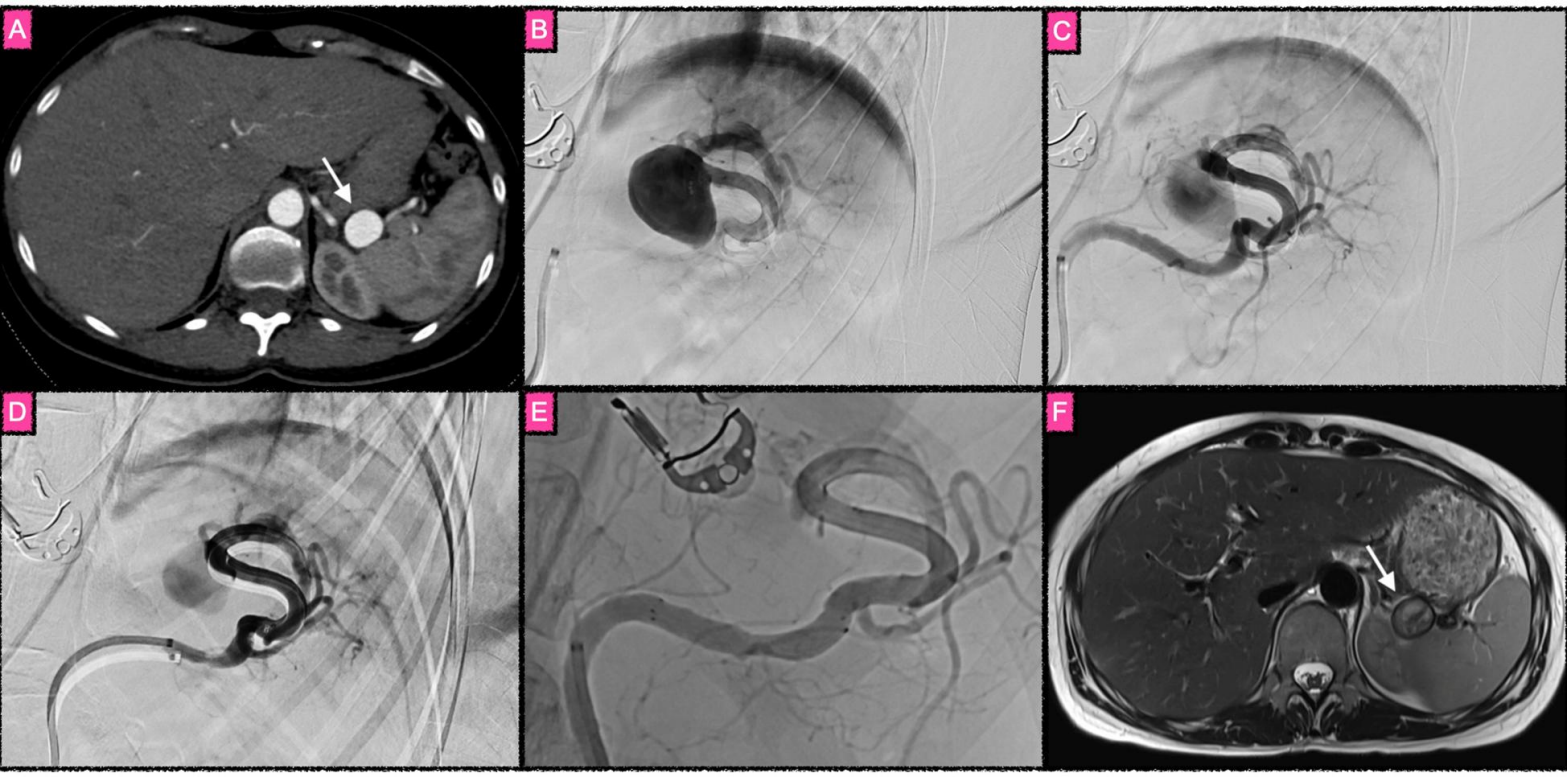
Treatment of a giant main stem aneurysm of the splenic artery with four overlapping flow diverter stents, equipped with antithrombogenic HEAL coating under prasugrel monotherapy. Given the large size of the aneurysm, the risk for delayed rupture after flow diversion–a rare but potentially fatal complication most likely occurring secondary to excessive thromboinflammation–was estimated to be comparatively high. Therefore, antiplatelet medication (prasugrel only) was reduced, based on the use of HEAL-coated FDS, which are significantly less thrombogenic and less proinflammatory. (A) Axial section of a computed tomography angiography in the arterial phase with the aneurysm (white arrow) in close proximity to the spleen. (B) Early phase of a superselective catheter angiography of the splenic artery after implantation of two FDS, with two adjacent FDS layers already covering the aneurysm neck. The DSA run reveals that there is still strong inflow into the aneurysm sac, whereas the splenic artery distal to the aneurysm is not as strongly opacified as the aneurysm itself. To further reduce aneurysm perfusion, two more FDS were implanted, so that a total of four layers of FDS covered the aneurysm neck. (C) DSA run of the splenic artery after the third FDS was implanted. (D) DSA run after the fourth FDS was implanted. In (C), the opacification of the aneurysm was already significantly decreased compared to (B); the reinforced hemodynamic effect of the fourth implant is visible in (D). Catheter manipulations and subsequent vasospasm caused a strong fishmouth-like deformation of the proximal landing zone of the FDS construct, resulting in a significantly impaired perfusion of the splenic artery. Therefore, a self-expanding laser-cut stent (Acclino Flex Plus) was implanted into the stenosing proximal landing zone, which resolved the issue. (E) Unsubstracted DSA run of the splenic artery with the final stent construct in place. Approximately 10 min after implantation of the four overlapping FDS, clot had formed that almost completely occluded the aneurysm, corresponding to an O'Kelly–Marotta scale grade C3. Note the regular opacification of covered side branches of the splenic artery in the presence of the FDS telescoping construct. (F) Follow-up MRI (T2weighted axial image) 3 months after the procedure revealed organized thrombus completely filling the splenic artery aneurysm (white arrow), with the artery regularly perfused (flow void distal to the aneurysm), and no splenic infarction observable.
